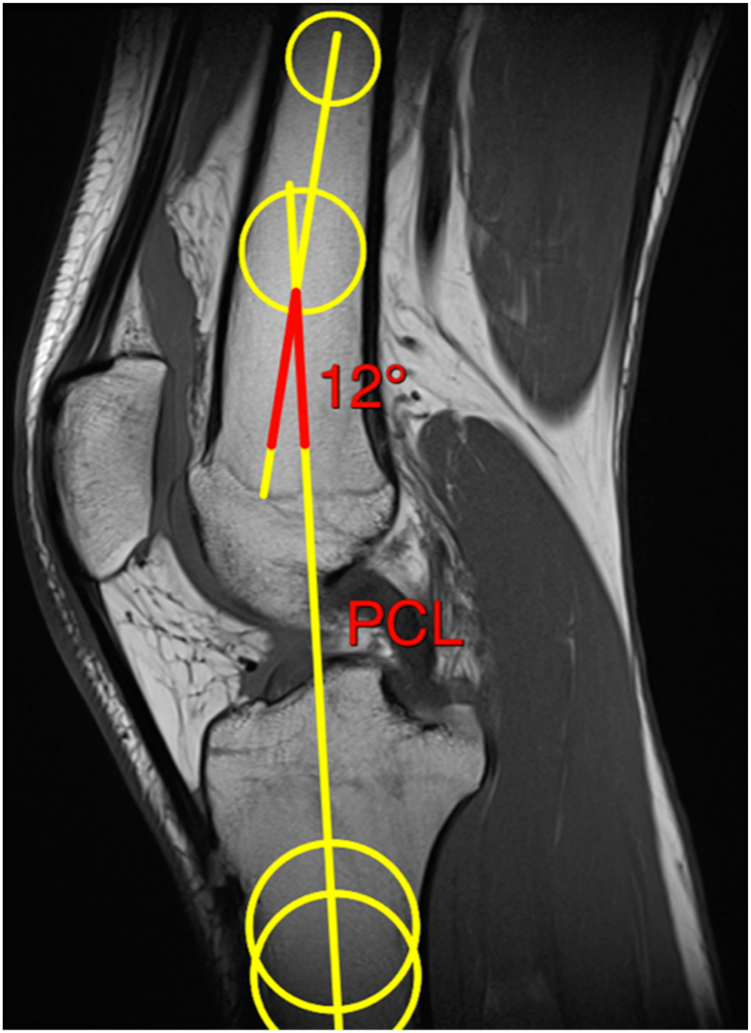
MRI flexion measurement. Sagittal T1‐weighted MRI images were used for the flexion measurement. The image slice was defined as the one on which the attachment of the PCL to the femur was visible. Two best‐fit circles were drawn in the subchondral portion of the femoral shaft, with the cortical bone of the femur serving as the limit of the circles. The first circle was placed as proximally as possible in the femoral shaft. The second circle was drawn in the distal part of the femoral shaft, whereby the center of this circle had to be at least 4 cm proximal to the femoral metaphysis. Next, two additional best‐fit circles were drawn in the proximal and distal parts of the tibia. The center points of these circles were connected by a line extending beyond them. The angle between the two resulting lines indicates the degree of flexion or extension of the knee joint. MRI, magnetic resonance imaging; PCL, posterior cruciate ligament.
